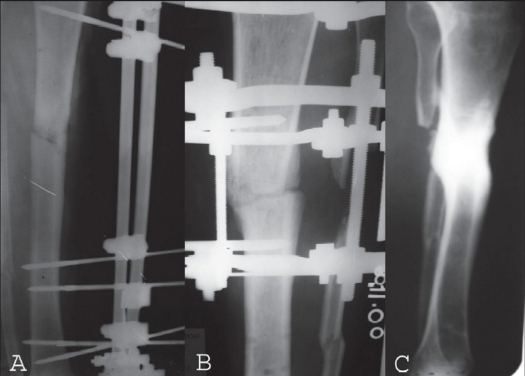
Hybrid assembly. A) Open tibial fracture treated initially with debridement and unilateral external fixator. B) X-ray depicting Hybrid assembly and early fracture union. C) X-ray post frame removal showing fracture consolidation
